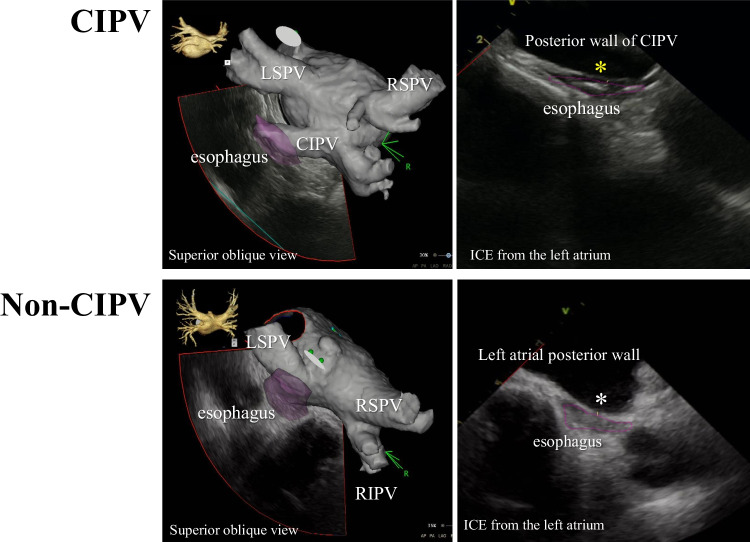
Representative intracardiac echocardiographic (ICE) images of the left atrium, pulmonary veins (PV), and esophagus in cases with confluent inferior pulmonary veins (CIPV) and with non-CIPV. The left panel show 3-dimensional (3D) computed tomographic images of the left atrium (superior oblique view) and ICE plane at the levels of CIPV and left inferior PV, revealing the thin wall of the posterior CIPV wall (yellow asterisk) and esophagus (right upper panel) and left atrial posterior wall (white asterisk) and esophagus (right lower panel). The 3D constructed esophagus is shown in purple in the left panels and the outline of the esophagus is drawn by a purple line in the right panels. LSPV, left superior pulmonary vein; RSPV, right superior pulmonary vein; RIPV, right inferior pulmonary vein
